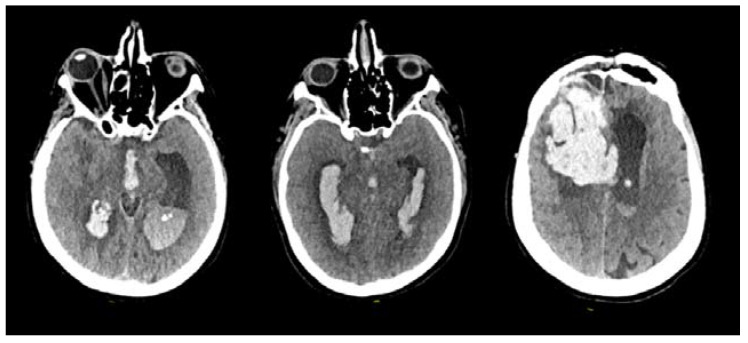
Initial CT scans of three different patients showing various intraventricular hemorrhagic extension patterns due to a ruptured bAVM.
